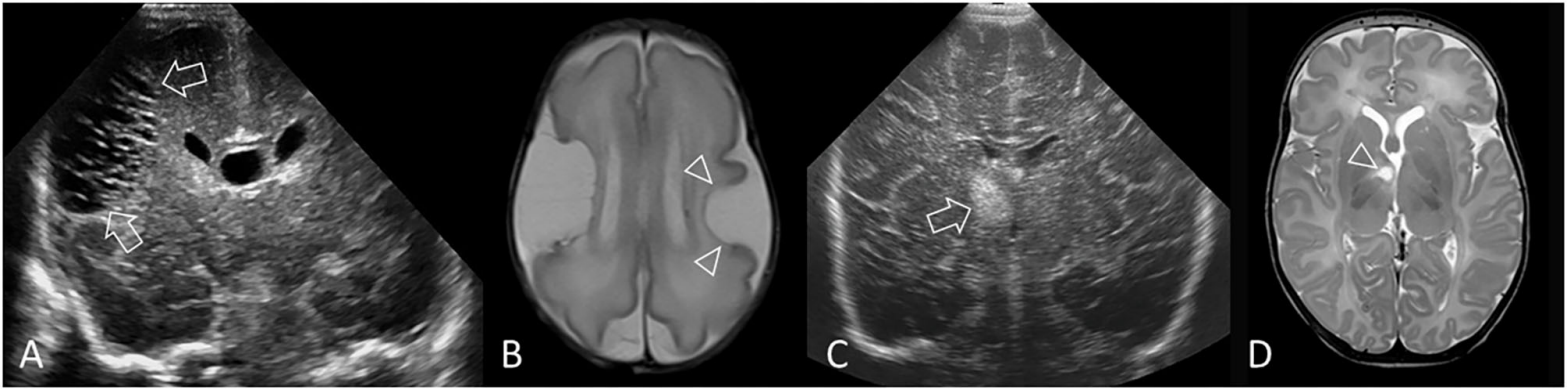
Other forms of acute injury. (A,B) Preterm infant (GA 27 weeks) with bilateral middle cerebral artery (MCA) territory infarction. (A) Coronal ultrasound scan 3 weeks after birth shows extensive cystic injury in the right frontoparietal region (arrows), but located more lateral than expected for common periventricular WMI. Focal echodensity was first identified at this location on cUS 7 days after birth (not shown). (B) Axial T2-weighted MR image shows the large right MCA cortical infarct and an additional smaller left MCA cortical infarct (arrowheads) that was not readily appreciated on cUS because of its far lateral location. The bilateral MCA strokes suggest a proximal embolic source, and placenta was suspected. (C,D) Perforator stroke in a preterm infant (GA 34 weeks). (C) Coronal ultrasound scan, at the level of the third ventricle and foramen of Monro, performed after surgery for ventriculoseptal defect closure shows a focal echodensity in the medial right capsuloganglionic region (arrow). cUS performed prior to surgery did not show any abnormality. Findings are most consistent with lenticulostriate territory infarction (middle cerebral artery perforating branches). (D) Axial T2-weighted MR image after 2 weeks shows cystic evolution of the infarct without evidence of previous hemorrhage (arrowhead).
